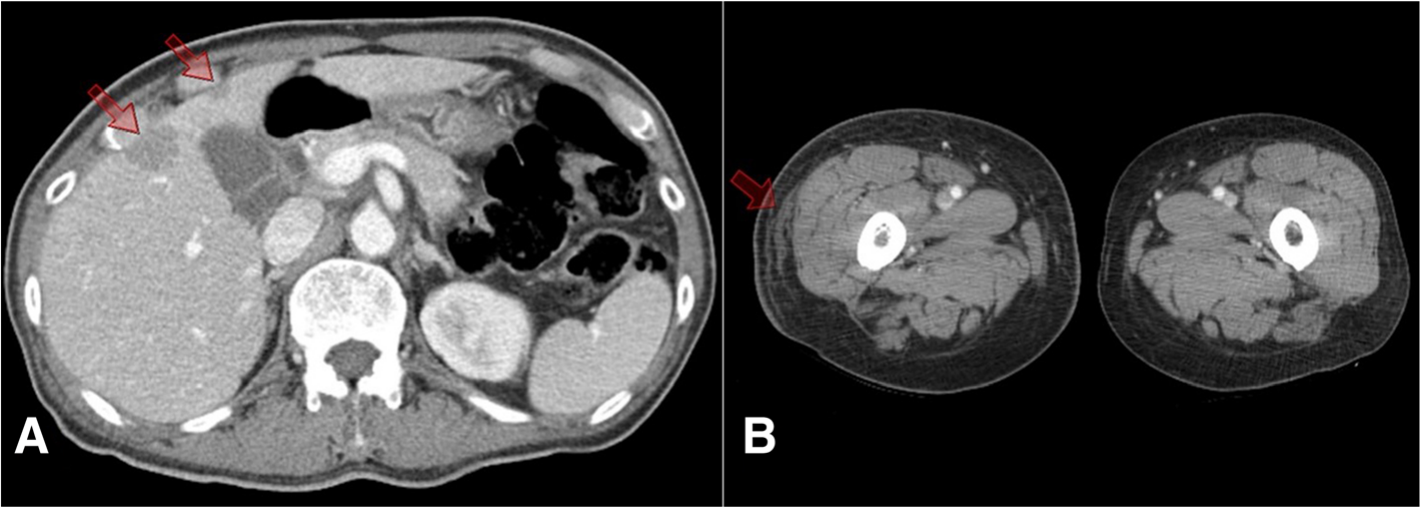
(a) CT of the abdomen revealed 2.5- and 1.5-cm low-attenuated lesions in liver segment 5, with other smaller lesions in both hepatic lobes. (b) Thigh CT showed edematous changes in subcutaneous tissues of the right thigh
